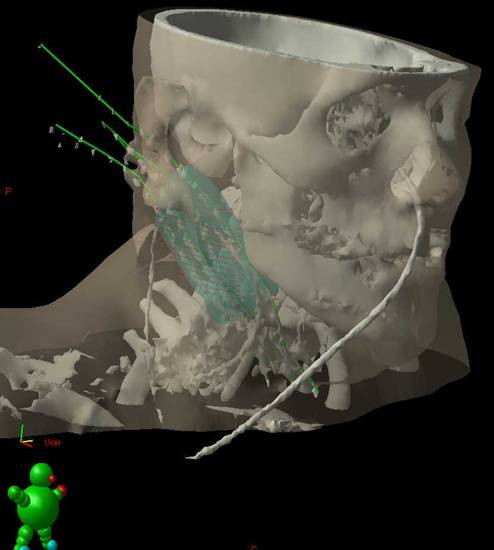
Three-dimensional planning CT image reconstruction.
